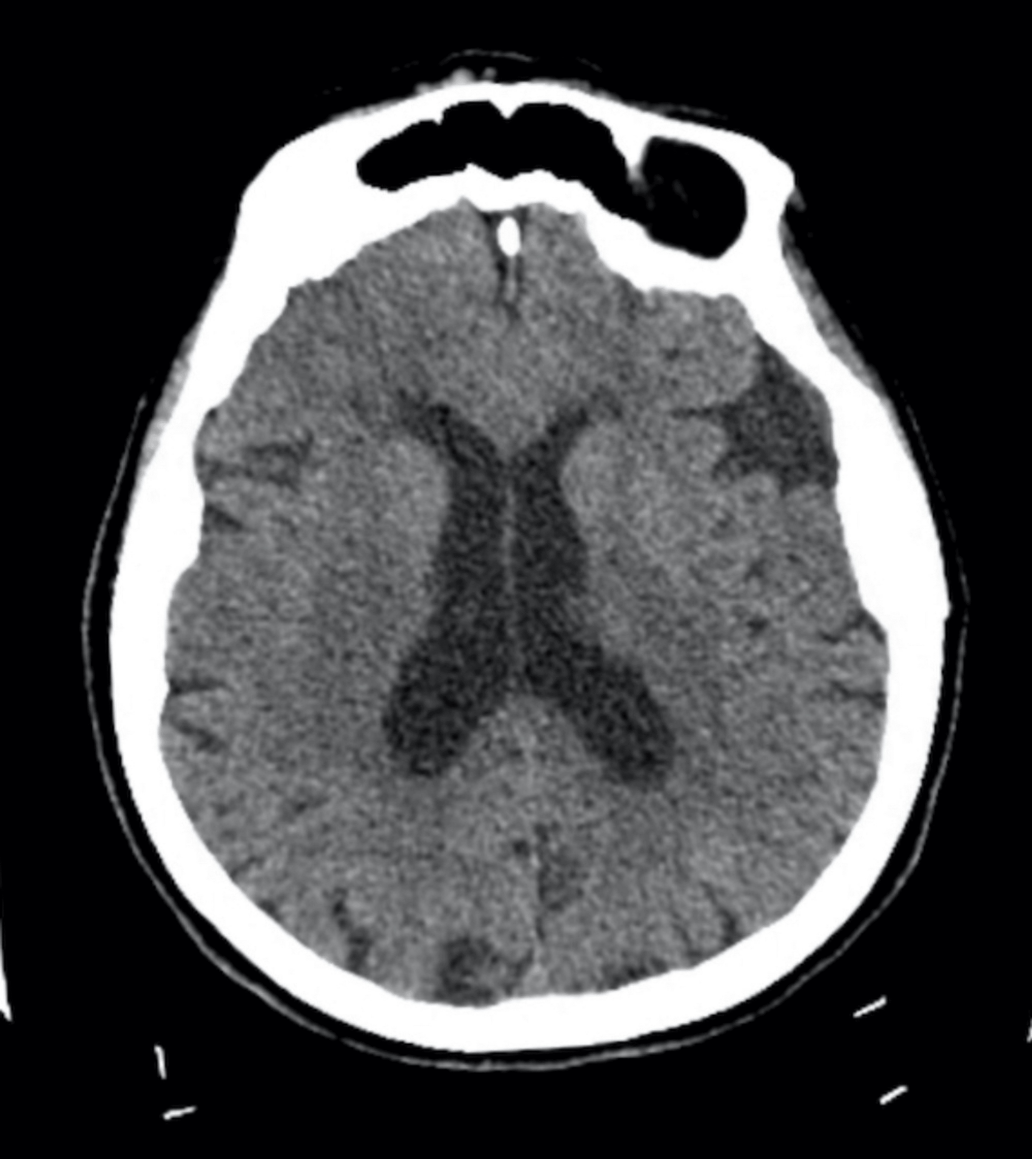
Non-contrast CT brain (axial) showing the absence of acute stroke CT: computed tomography
